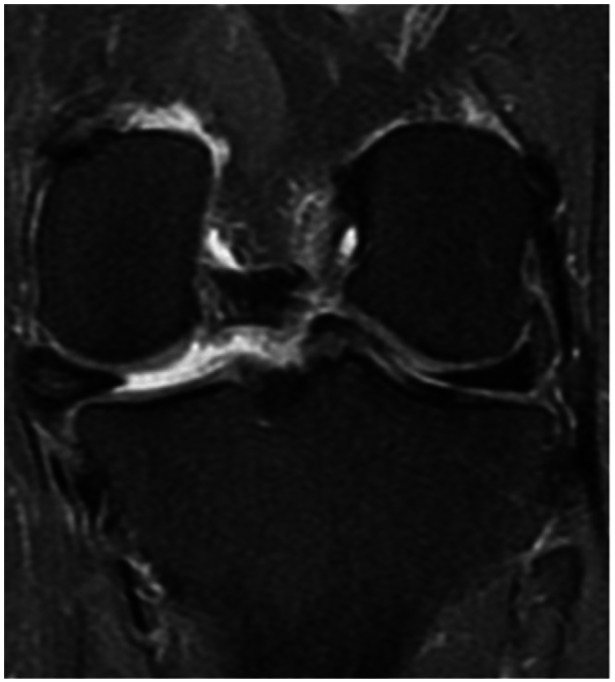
MRI coronal view of a left knee. Posterior medial root tear lesion associated with meniscal extrusion. MRI, magnetic resonance imaging.
